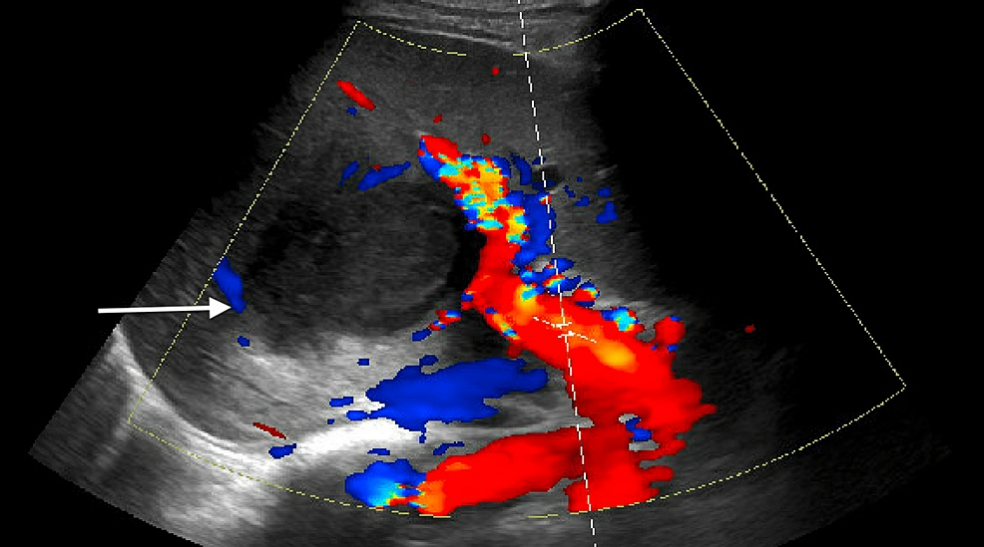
Right upper quadrant ultrasound Right upper quadrant ultrasound showing a mildly vascular lobulated hypoechoic mass in the right hepatic lobe measuring 11.7 x 9.7 cm.
